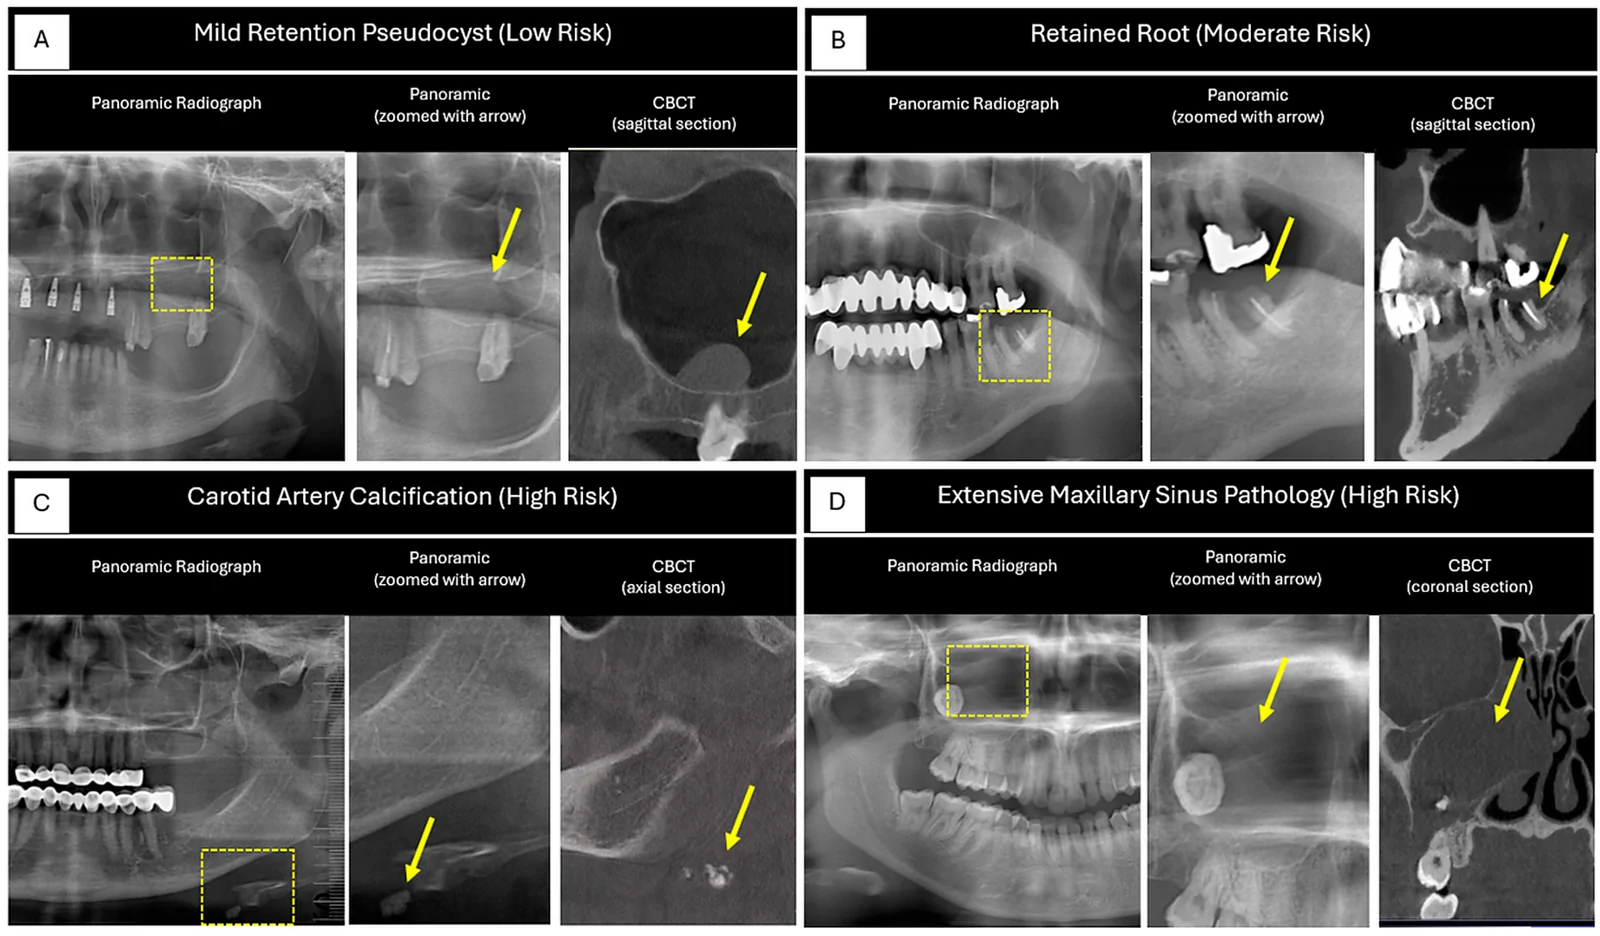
Representative CBCT-confirmed incidental findings included in the study. (A) mild retention pseudocyst, (B) residual root, (C) carotid artery calcification, and (D) extensive maxillary sinus pathology. For each lesion, the corresponding panoramic radiographic image, a magnified panoramic view, and the relevant CBCT sections are shown. Dashed lines and arrows indicate the lesion sites. CBCT: cone-beam computed tomography.
